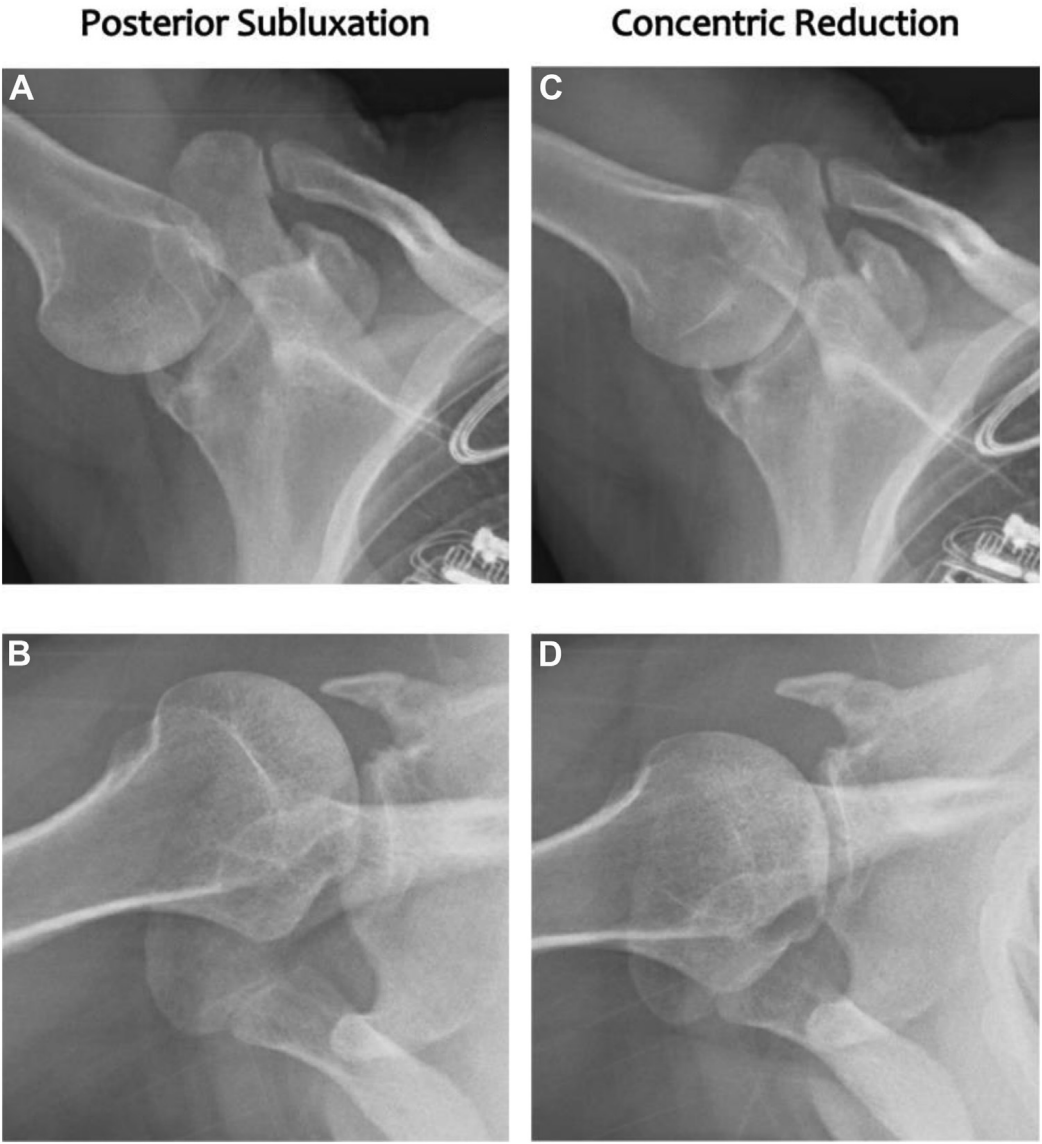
Static images of the digital dynamic radiography of the left glenohumeral joint. (A) Demonstrates a PA L shoulder XR and (B) an axillary view showing posterior subluxation. (C and D) With slight adduction, the humeral head concentrically relocates on corresponding views. *PA*, postero-antero; *L*, Lateral; *XR*, X-rays.
